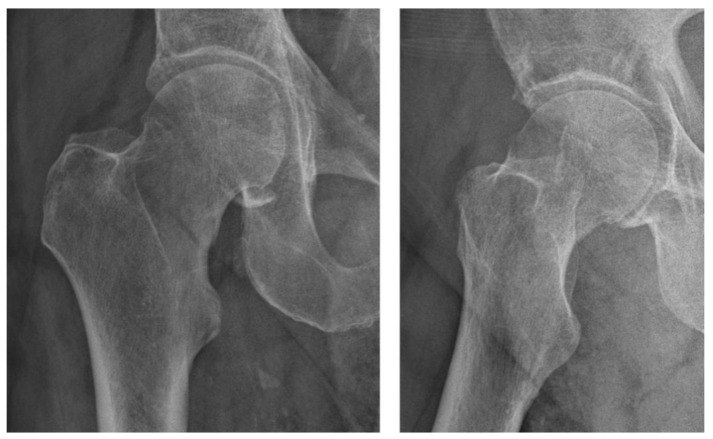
Plain radiograph of the right hip showing no clear signs of a fracture. The doctor then performed an X-ray imaging of the hip, which did not give clear signs of a fracture. Occult fractures are a type of nondisplaced fracture, which are not visible on plain radiographs or, sometimes, computed tomography (CT). This makes them prone to diagnostic errors, requiring a detailed anamnesis and clinical examination. The prevalence of such fractures is relatively high among children [10], whereas in adults the prevalence is under 10% [11,12]. An occult fracture should always be suspected when clinical symptoms and examination do not match the radiographic finding [12]. After X-ray, the next diagnostic step is the CT, which does not always exclude a false negative result. In addition to plain radiographs and CT, magnetic resonance imaging (MRI) plays an important role. Unfortunately, facilities equipped with MRI scanners are not available nationwide in all countries, and the cost–benefit has not yet been proven [13].
